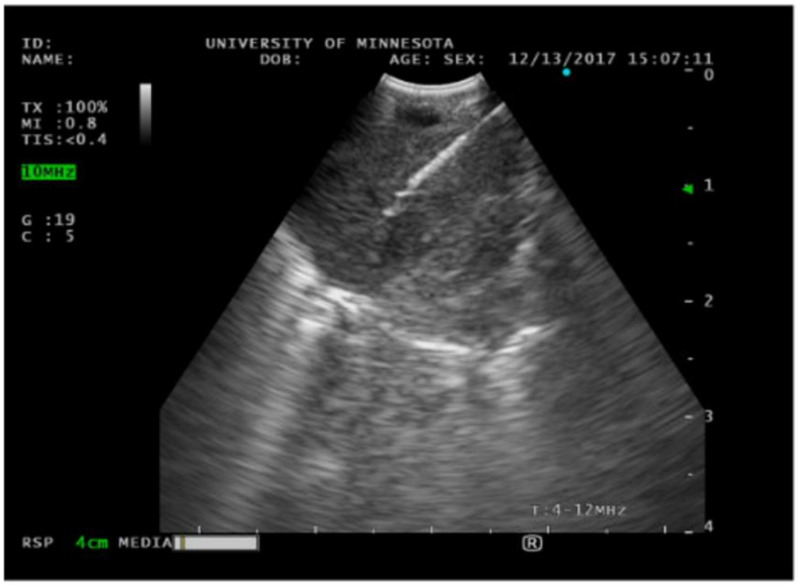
Endobronchial ultrasound image of a central lung mass. A needle is observed performing fine-needle aspiration.
